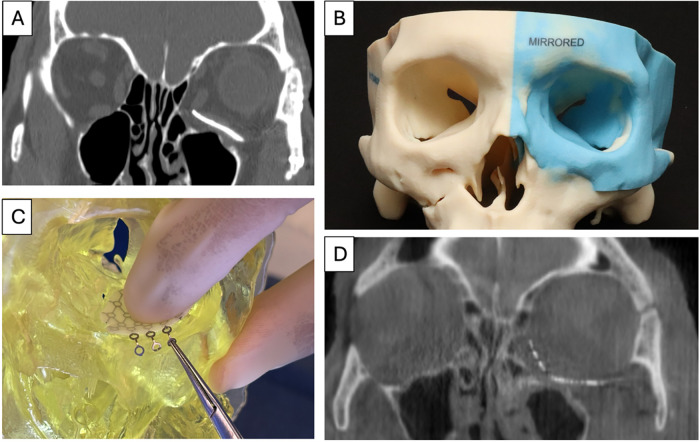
Correction of undersized hardware using 3D models. (A) Previously placed implant is not fully correcting the large orbital floor and medial wall fracture; the patient had 5-mm residual enophthalmos. (B) 3D model with mirror-image overlay of unaffected right side onto the left orbit. (C) Intraoperative shaping and molding of standard MEDPOR titan orbital floor implant on a sterilized mirror-image model. (D) Intraoperative CT demonstrates adequate implant placement and correction of orbital deformity with restored symmetry.
